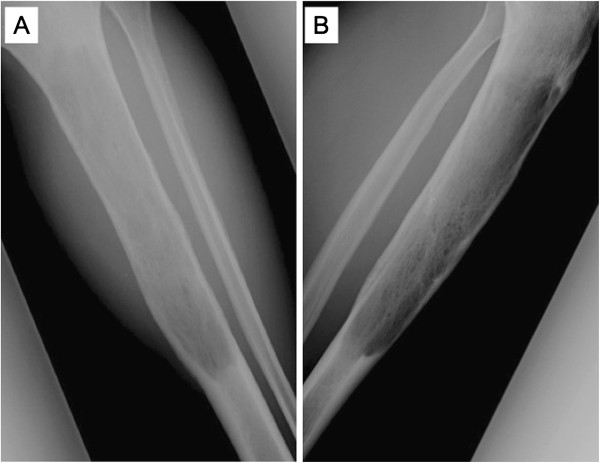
Case 1: A) Frontal and B) lateral radiographs of the tibia showing an expansile permeative lytic lesion involving the proximal tibial diaphysis.
